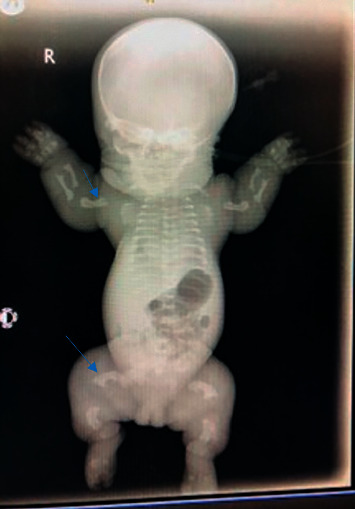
Plain radiograph of baby 1 with features of TD type 1. Arrows show telephone-handle shaped humerus and femur.
